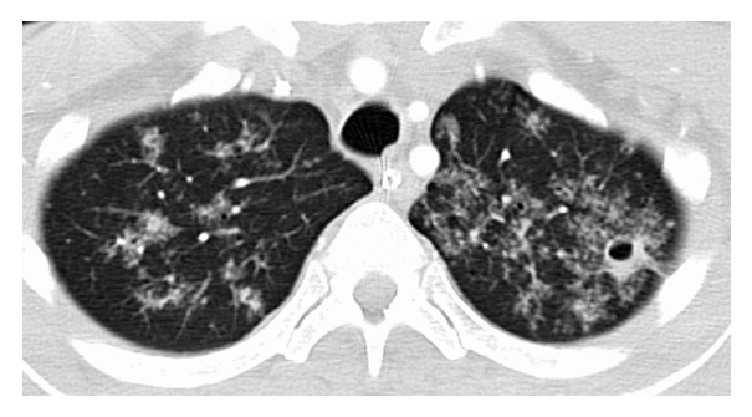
Chest CT scan showing diffuse airspace disease with associated reticular nodular opacities and apparent tree in bud configuration with small cavitary pulmonary nodule noted at the superolateral left lung apex.
